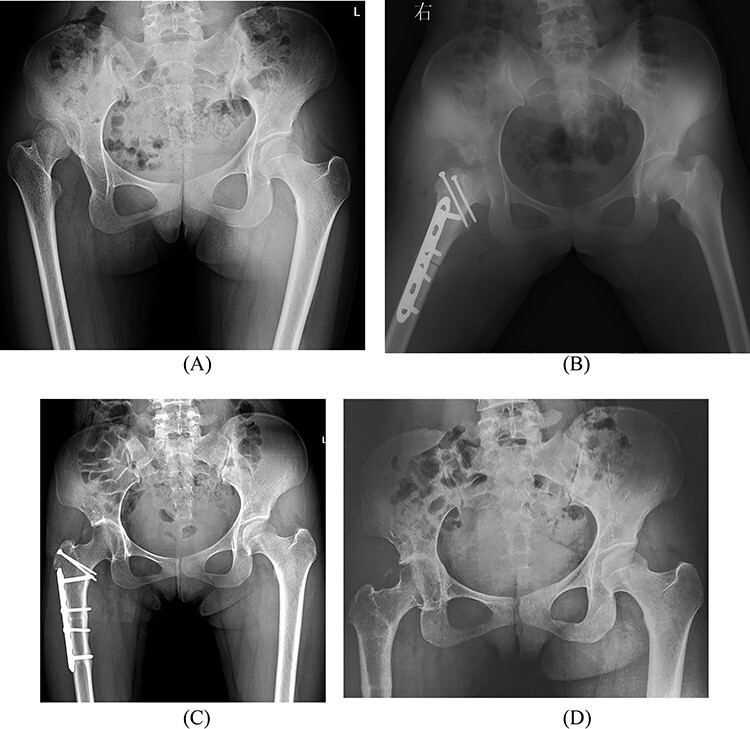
The modified capsular arthroplasty combined with proximal femoral derotation and shortening osteotomy in a 20-year-old female with a right hip dislocation (Hartofilakidis Type III). (A) Pre-operative radiograph; (B) post-operative radiograph; (C) 3 years post-operatively and (D) 6 years and 5 months post-operatively. The final radiograph shows the joint space is normal.
